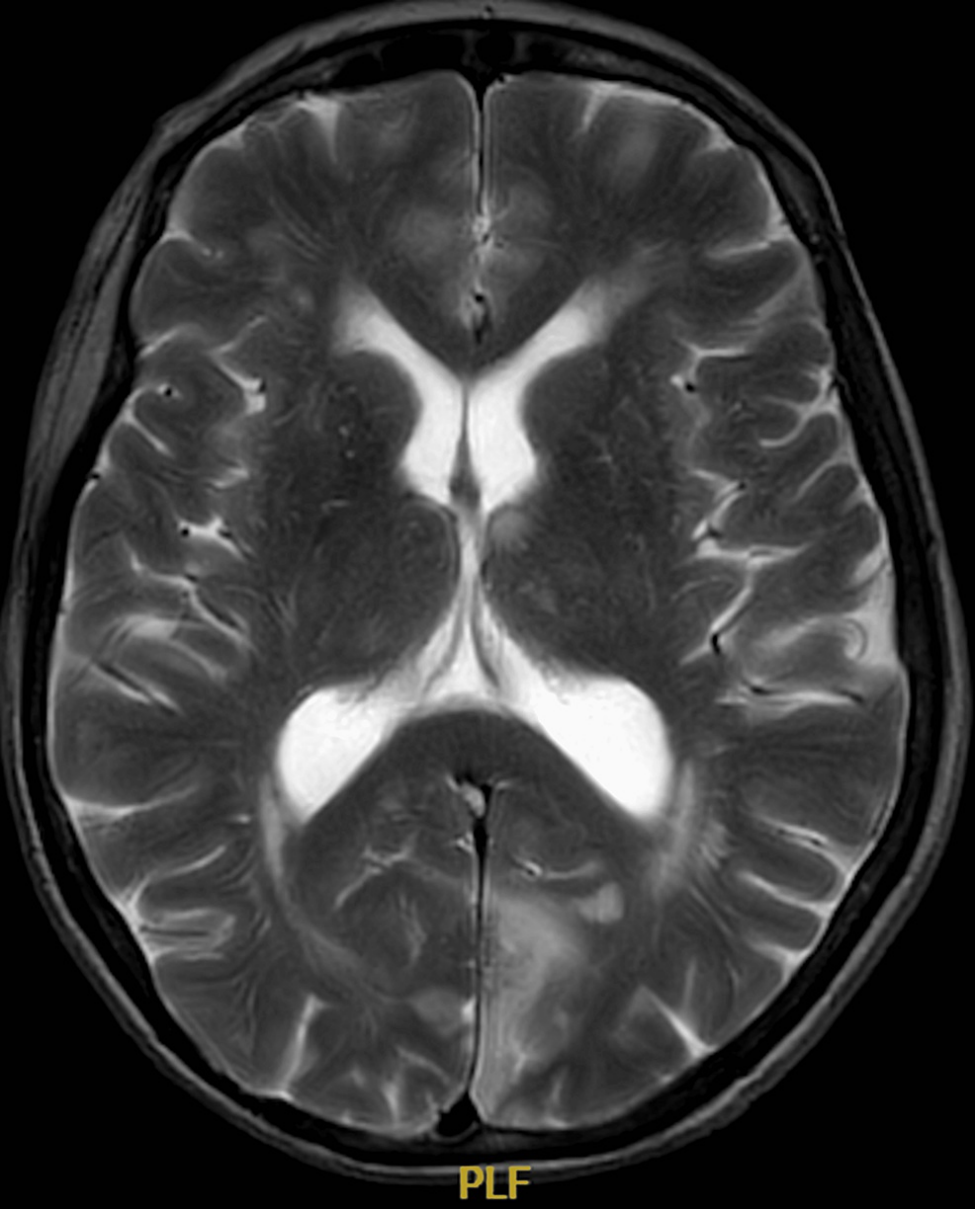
An axial, T2 magnetic resonance image showing extensive bilateral posterior cerebral artery territory infarcts involving the medial occipital lobes and the basal ganglia and thalamus region.
